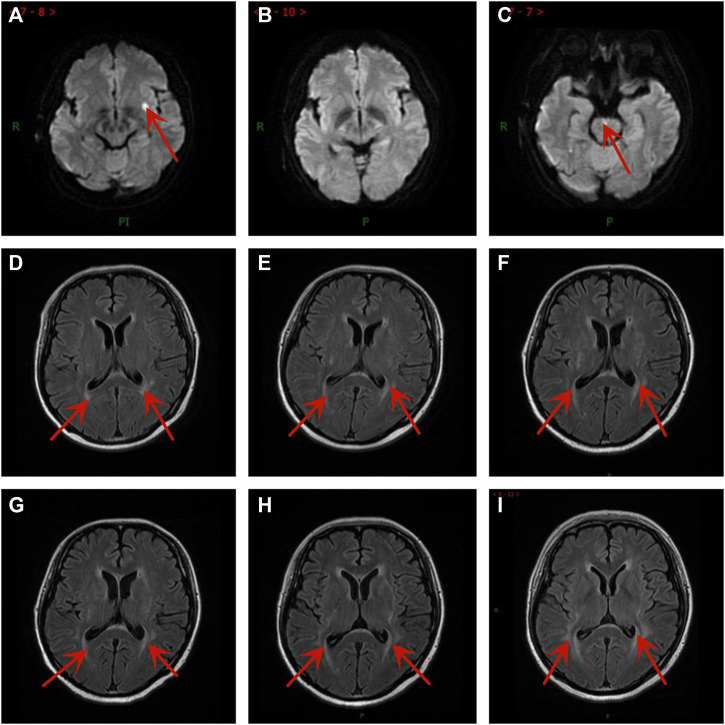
Brain magnetic resonance imaging images at different time points. (A) Brain metastases were newly diagnosed after second-line treatment. (B) One month after brain radiation therapy. (C) New brain metastasis after taking anlotinib 10 cycles. The PRES developed gradually after taking anlotinib for (D) 1 cycle, (E) 3 cycles, (F) 4 cycles, (G) 6 cycles, (H) 8 cycles, and (I) 10 cycles.
